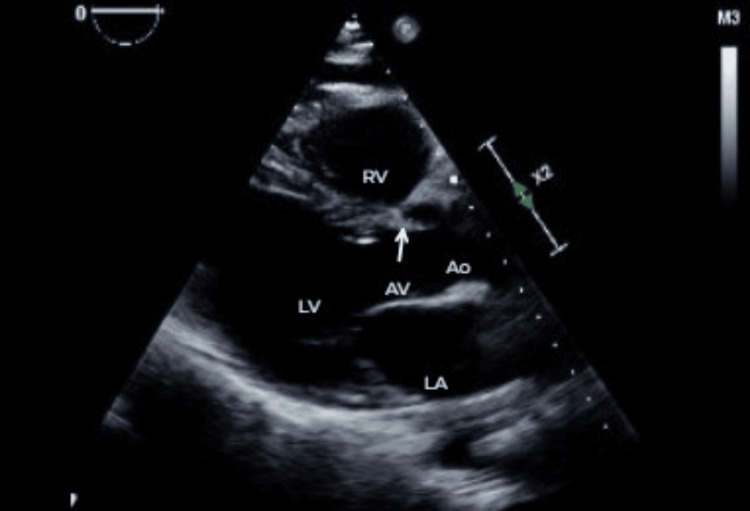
Transthoracic echocardiogram: Parasternal long-axis (PLAX) view demonstrating no obvious vegetations. RV: right ventricle; Ao: ascending aorta; AV: aortic valve; LV: left ventricle; LA: left atrium
